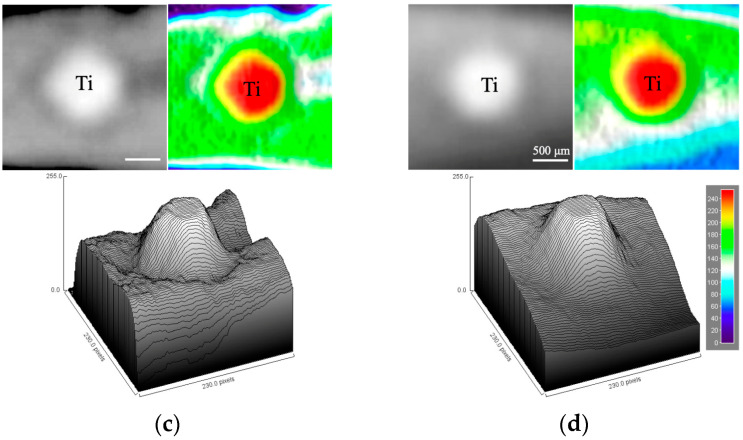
Soft X-ray images of peri-implant bone: (a) 14 days post-implantation under atmospheric storage; (b) 14 days post-implantation with NBW3 storage; (c) 28 days post-implantation under atmospheric storage; (d) 28 days post-implantation with NBW3 storage. “Ti” denotes the titanium implant.
